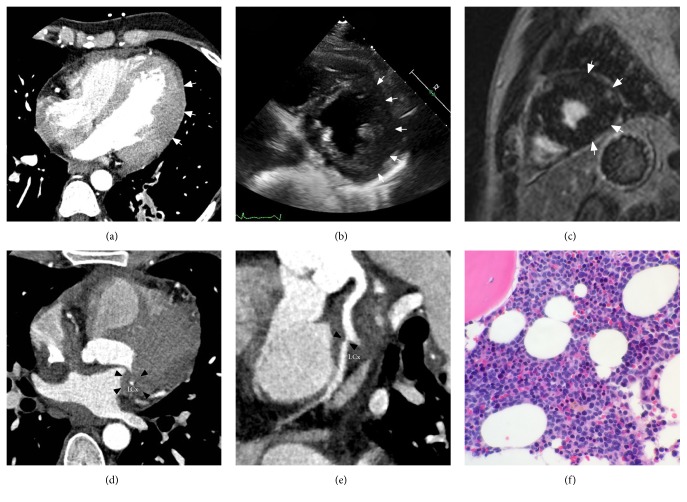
(a) Axial coronary computed tomography angiogram (CCTA), (b) echocardiogram, and (c) CMR with delayed myocardial enhancement images demonstrate marked asymmetric thickening of the lateral left ventricular wall* (arrows)*. There is obliteration of left ventricular pericardial fat on CCTA. (d) Axial and (e) curviplanar reformatted CT images of the left circumflex artery* (LCx)* show abnormal soft tissue in the left atrioventricular groove* (arrowheads)* resulting in approximately 50% stenosis of the LCx immediately after the takeoff of the first obtuse marginal branch. (f) Bone marrow biopsy slide demonstrates replacement of normal hematopoietic elements by small lymphoblasts comprising approximately 50% of total cellularity, compatible with leukemia recurrence.
